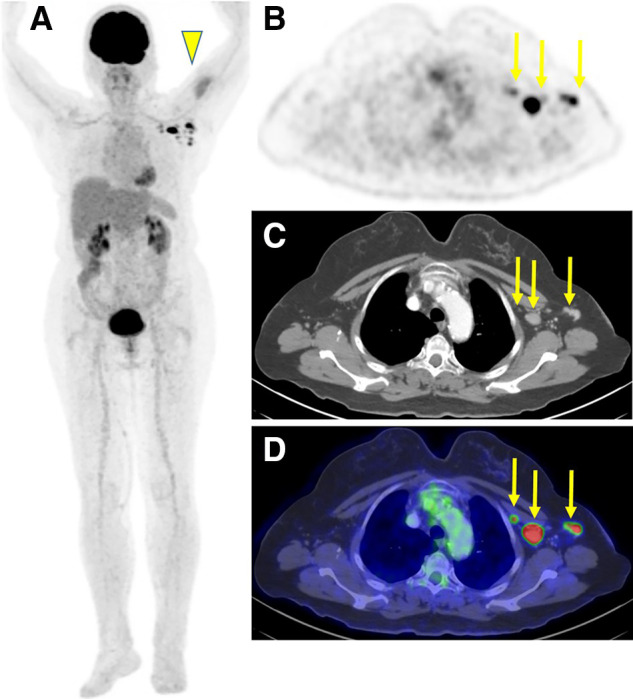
^18^F-FDG PET/CT after COVID-19 vaccination in 66-y-old woman with suspected colon cancer and elevated carcinoembryonic antigen, with no current immunosuppressive treatment: maximal-intensity projection (A), axial multiplanar reformation (B), contrast-enhanced CT (C), and PET/CT (D) 25 d after first vaccine dose and 2 d after second vaccine dose, both in left arm. Increased uptake was observed in left deltoid muscle (arrowhead), corresponding to injection site, and in ipsilateral enlarged axillary lymph nodes (arrows). Otherwise, there were no hypermetabolic findings suggestive of malignancy.
